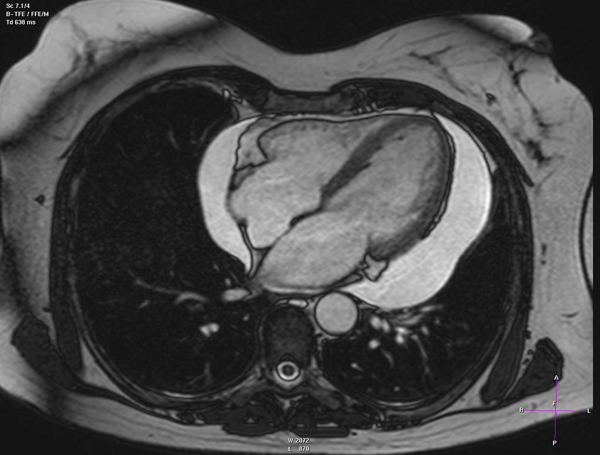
Magnetic resonance imaging (4-chamber view, turbo field echo [TFE]) confirmed the extended pericardial effusion without signs of cardiac tamponade, and a slightly thickened pericardium (see SD3 and SD4).
